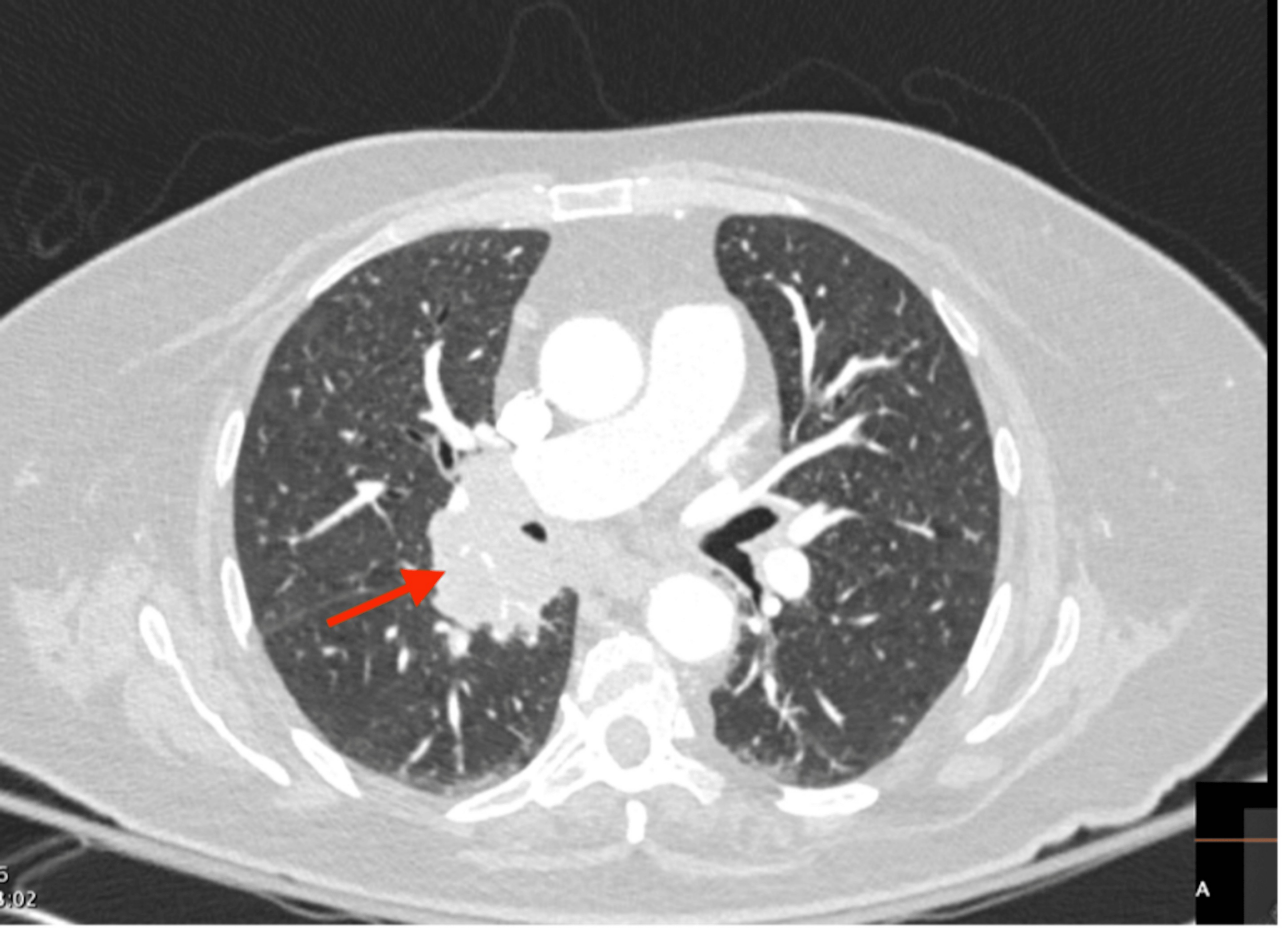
CT angiography of the chest showing a soft tissue mediastinal mass measuring 4.8 x 3.7 x 3.7 cm (red arrow)
